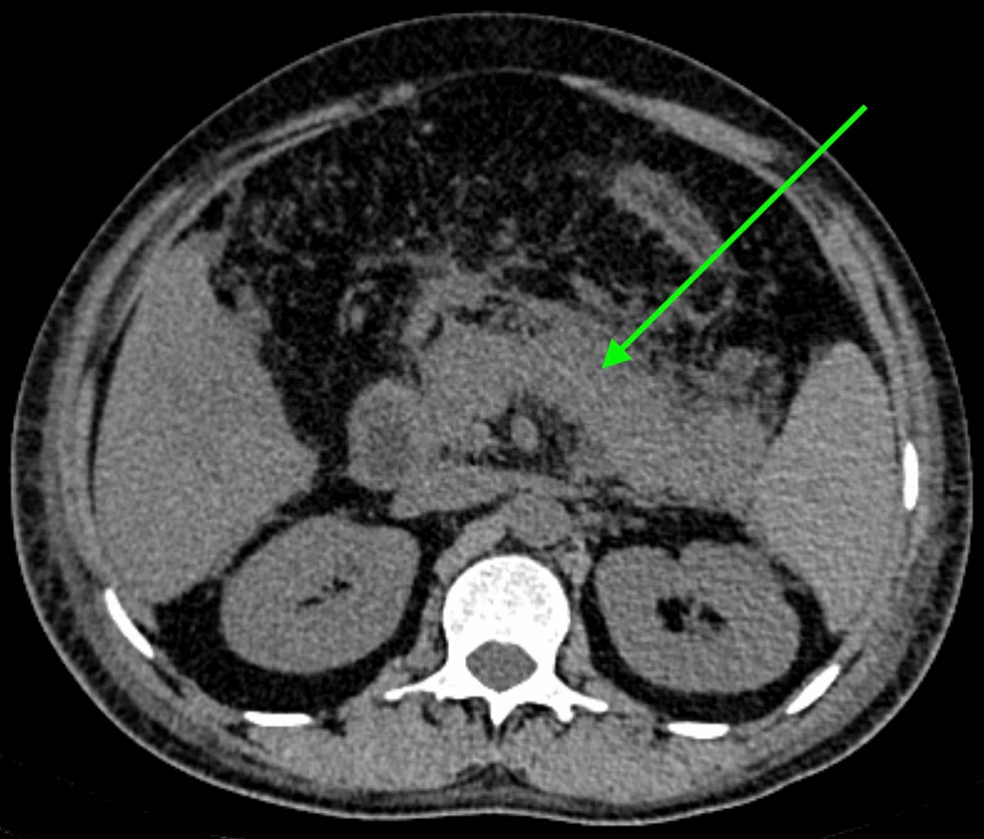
Non-contrast computed tomography of the abdomen of the patient with findings suggestive of acute pancreatitis (green arrow points toward the inflamed pancreas as seen on computed tomography)
